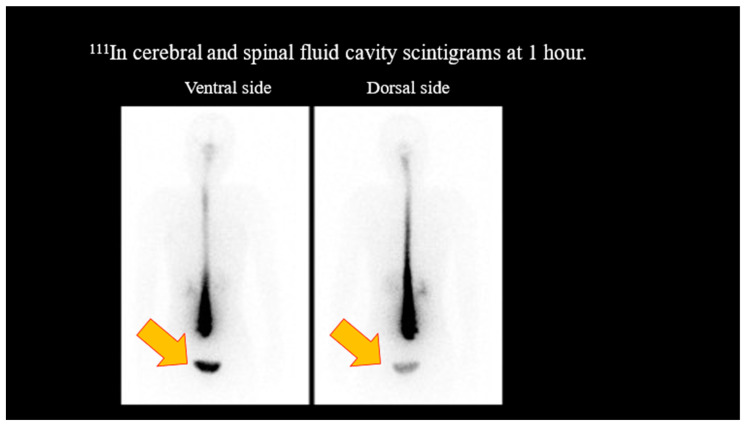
^111^In cerebrospinal fluid cavity scintigrams at 1 h. No spinal fluid leakage was observed. However, early accumulation of radioisotopes in the bladder was observed, suggesting spinal fluid leakage.
